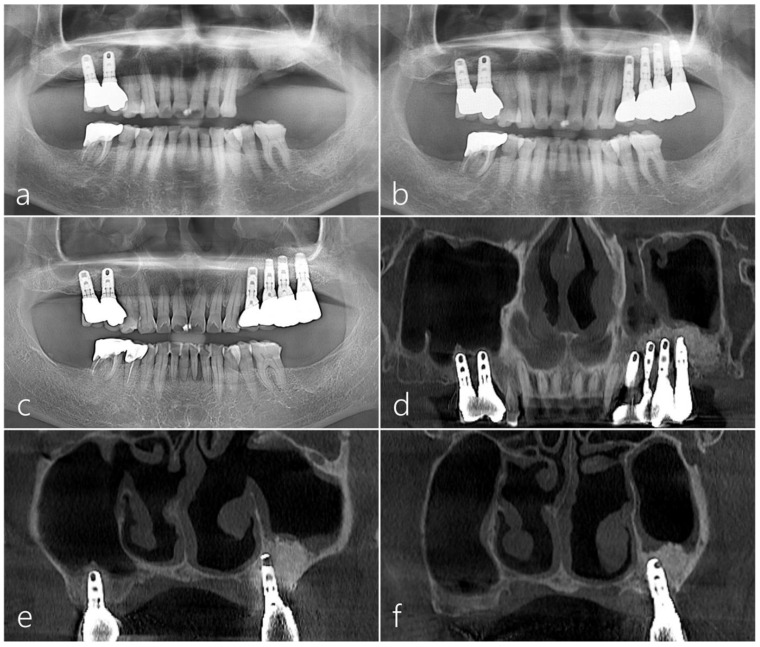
(a) Panoramic radiography taken immediately after maxillary sinus augmentation (MSA) using a particulate bone graft and a cortical bone shell showed radiopaque bone density in the left maxillary sinus. (b) Four implants were placed 6 months after MSA, followed by the delivery of the prosthesis 6 months after the implant placement. (c) Panoramic radiography 5 years after the delivery of the prosthesis. (d–f) On the panoramic and coronal images of the CBCT taken 5 years after the prosthesis was delivered, the left sinus showed no significant mucosal thickening and maintained bone integrity.
